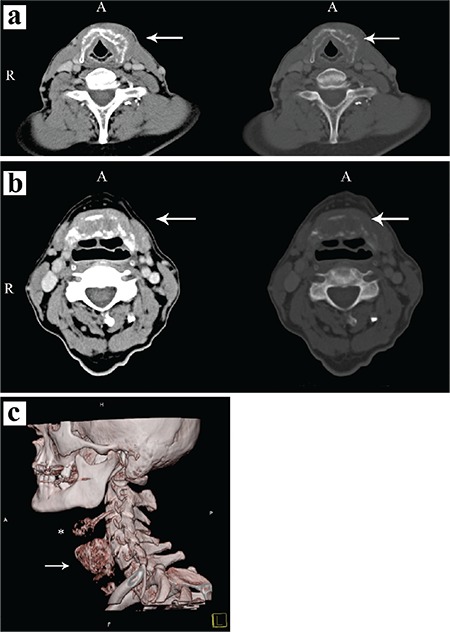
Contrast-enhanced CT scans of the neck: (a) Enlargement and osteolyses of the hyoid bone (arrows) presented in a soft tissue and bone window (A-anterior, R-right); (b) Tumour mass in the thyroid cartilage with reactive sclerosis (arrows) presented in a soft tissue and bone window (A-anterior, R-right); (c) 3D reconstruction image of thyroid cartilage (arrow) and hyoid bone (asterisk) defects (A-anterior, H-head, P-posterior, F-foot).
